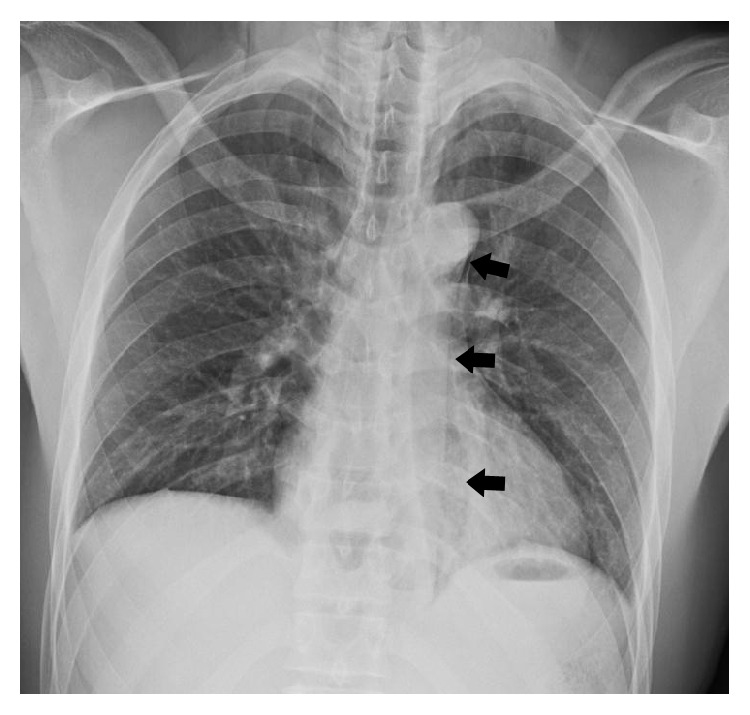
Chest X-ray displaying pneumomediastinum (indicated by black arrows) and left-sided pleural effusion.
